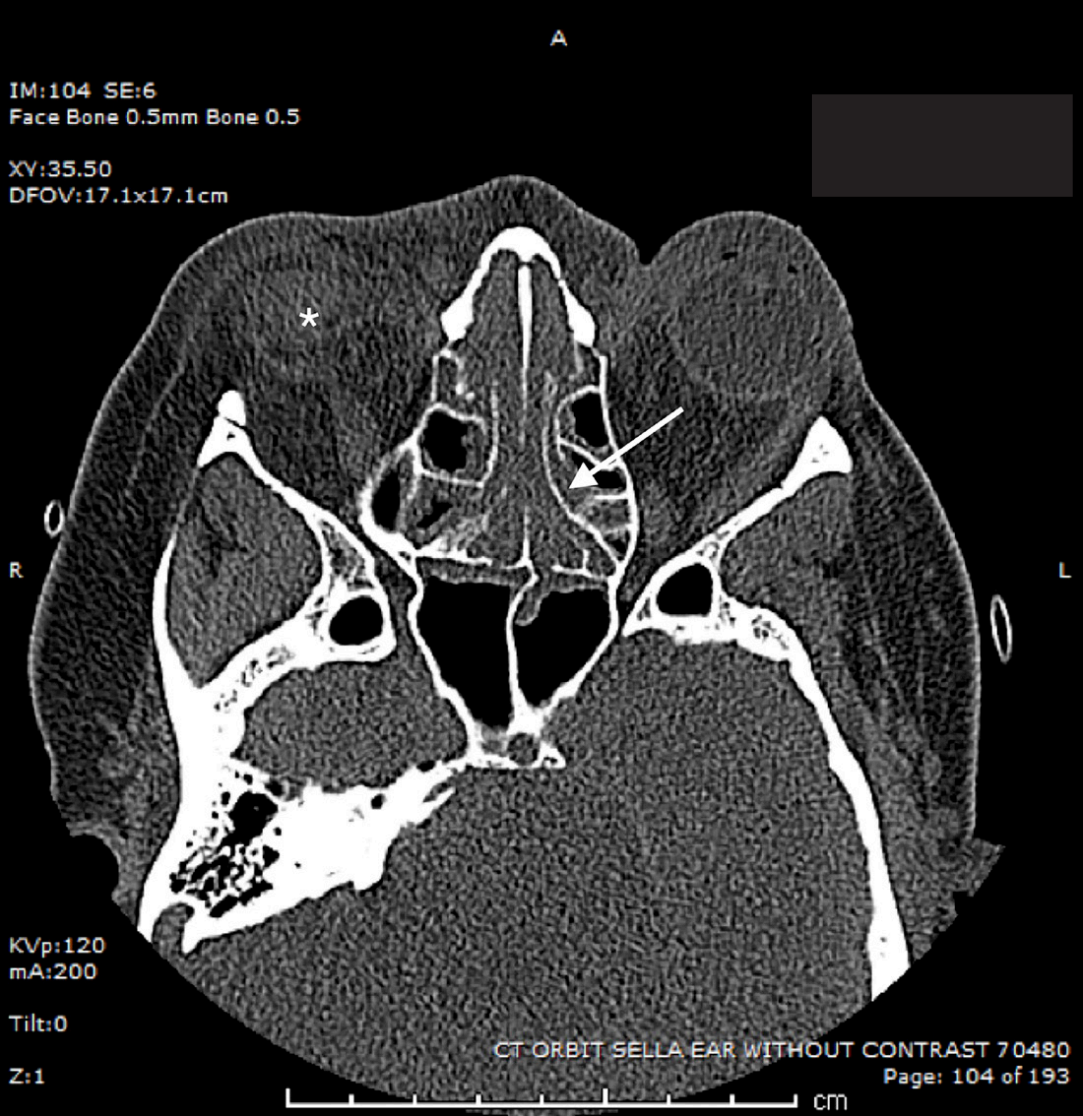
Computed tomography of the orbits, sella and ears without contrast. Right periorbital soft tissue swelling (*), and mucosal thickening within all the paranasal sinuses (arrow).
